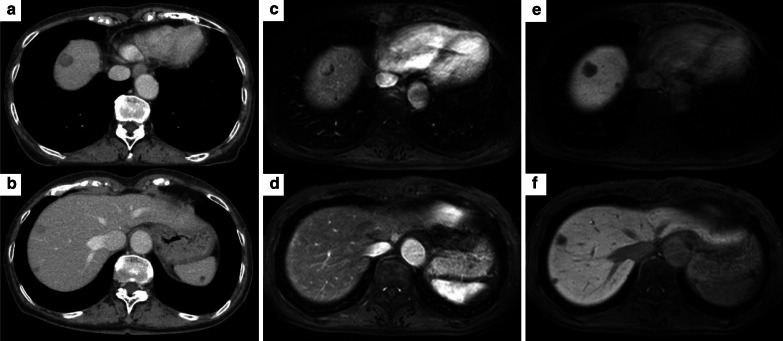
a, b CT image. Four tumors are seen in liver segments S7 and S8. c–f EOB-MRI image. The tumor edges are hyperintense with dynamic imaging, and some enhancement is exhibited within the tumors
